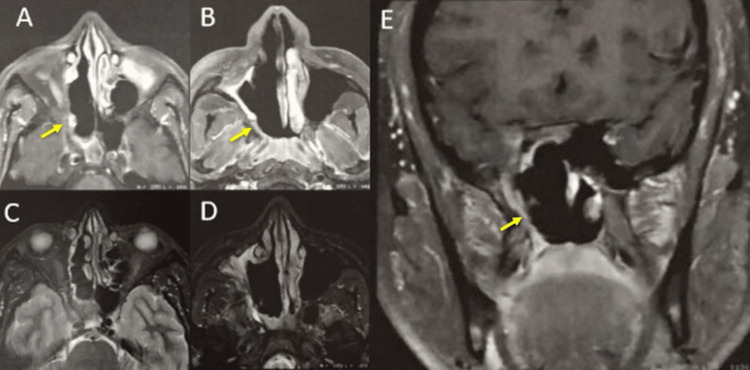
MRI following surgical intervention A partial right ethmoidectomy and right turbinectomy were performed, including the surgical excision of a cavity connecting the nasal fossa and right ethmoid cells as well as the right maxillary sinus. Upon examination, we observed an enlarged right choana, as depicted by the yellow arrows in images 5A, 5B, and 5E. T2-weighted axial images 5C and 5D strongly imply a gross total resection of the tumor. MRI: magnetic resonance imaging
